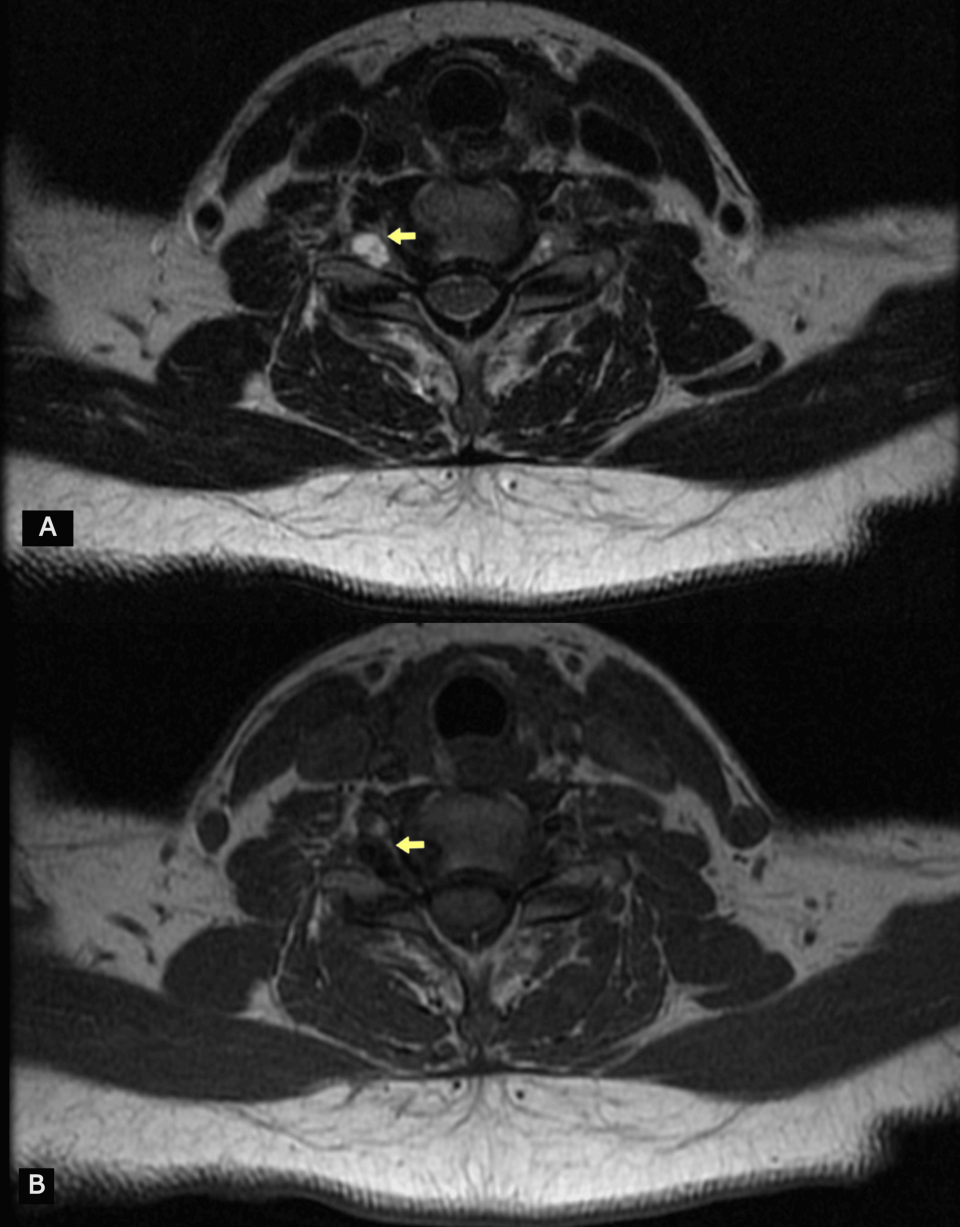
Magnetic resonance image of the cervical spine. Axial images (T2-weighted (A) and T1-weighted (B)) revealing the presence of a perineural cyst at the right neural foramen formed by C6 and C7 vertebra. The perineural cyst (arrow) is hypointense on the T1-weighted image and hyperintense on the T2-weighted image.
